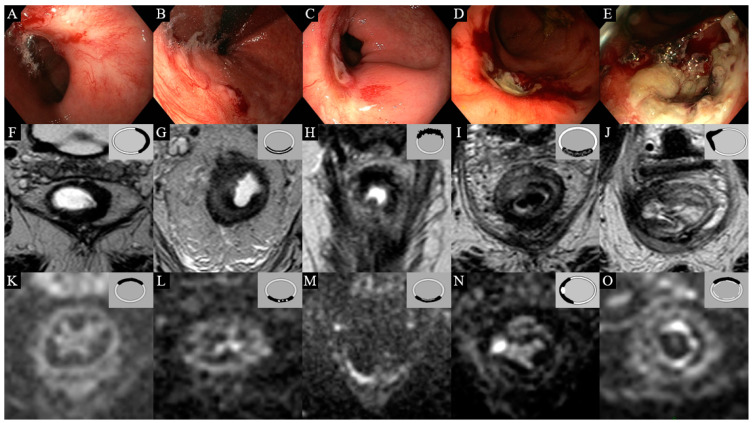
Features on endoscopy, T2W-MRI, and DWI. (A) Flat, white scar on endoscopy; (B) adenomatous tissue on endoscopy; (C) ulcer with regular borders on endoscopy; (D) ulcer with irregular borders on endoscopy; (E) tumor mass on endoscopy; (F) regular homogeneous fibrosis on T2W-MRI; (G) layered fibrosis on T2W-MRI; (H) irregular fibrosis on T2W-MRI; (I) heterogeneous fibrosis on T2W-MRI; (J) ulceration on T2W-MRI; (K) no high signal on DWI; (L) small spots of high signal on DWI; (M) linear high signal on DWI; (N) mass-like high signal on DWI; (O) a diffuse “reactive” mucosal diffusion signal on DWI.
